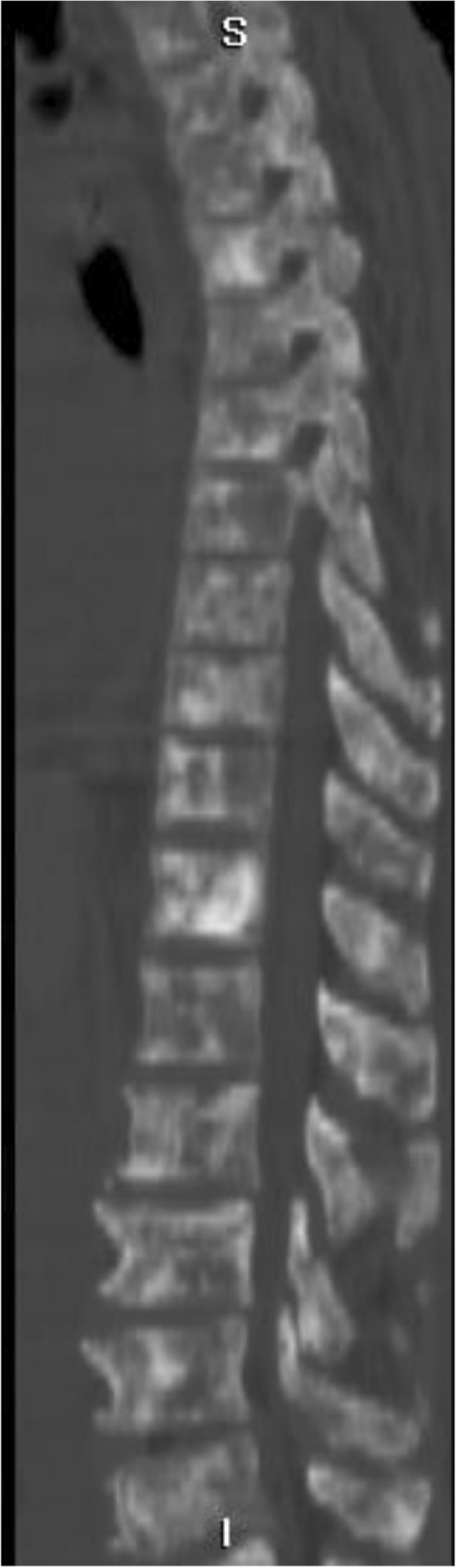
Computed tomography scan showing lytic lesions involving the entire spine.
